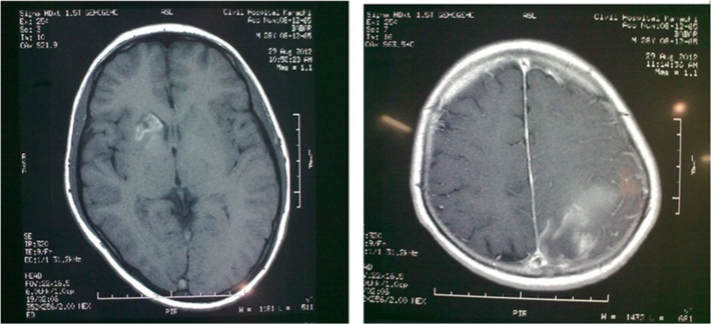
Magnetic Resonance Imaging (MRI) brain revealing multiple hemorrhagic infarcts.
